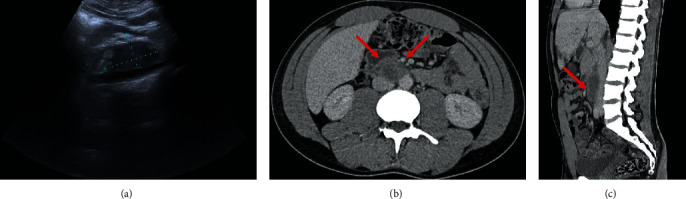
Ultrasound and CT. (a) Sagittal gray-scale abdominal ultrasound of the superior retroperitoneum, demonstrating a hypoechoic enlarged lymph node (between calipers). (b, c) Axial and sagittal contrast-enhanced CT abdominopelvic images showing the same enlarged lymph node (red arrows), on staging. Note the discrete fat stranding in the adjacent planes.
